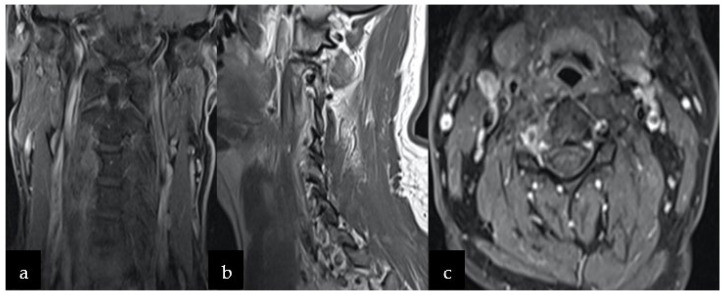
Follow-up MRI after 12 months showing no signs of recurrence on T-weighted 1 sequence with contrast in the following planes (a) coronal, (b) sagittal, and (c) axial.
